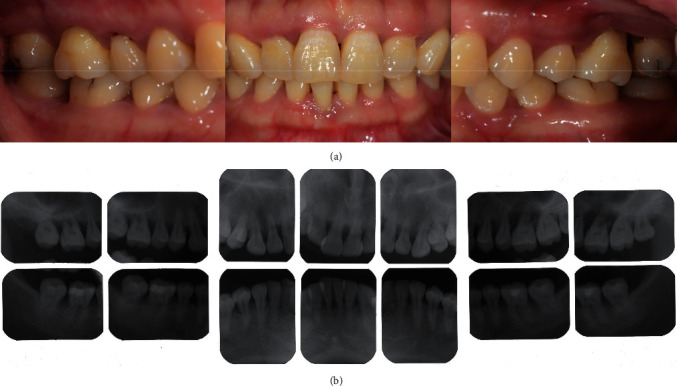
The patient had been diagnosed with aggressive periodontitis 4 years ago. Teeth #16 and #26 were severely compromised with bone loss to the apical third of root. (a) Intraoral photographs. (b) Periapical radiographs.
